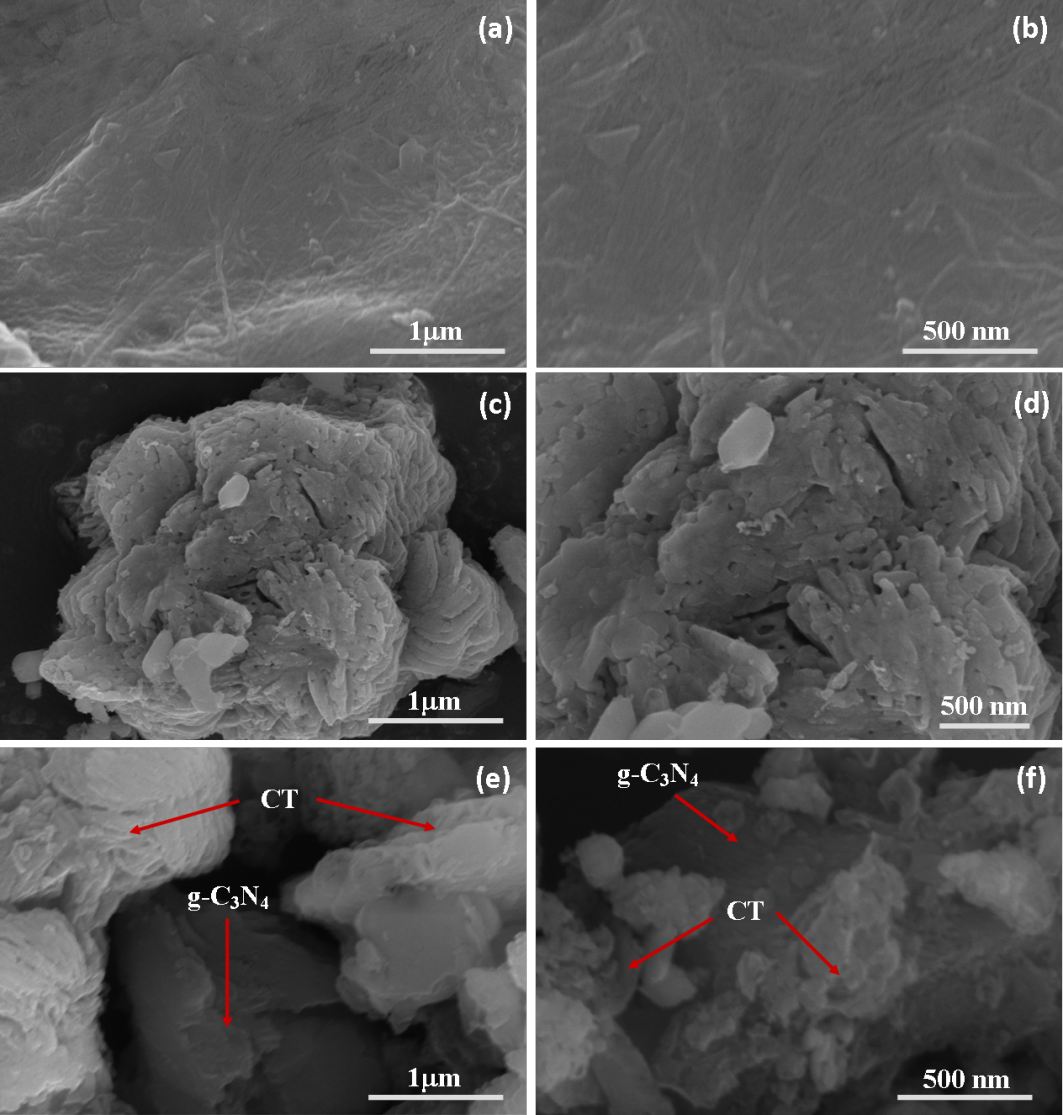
SEM images of (a, b) g-C_3_N_4_ sheets, (c, d) CT flakes and (e, f) CTCN heterojunctions.
